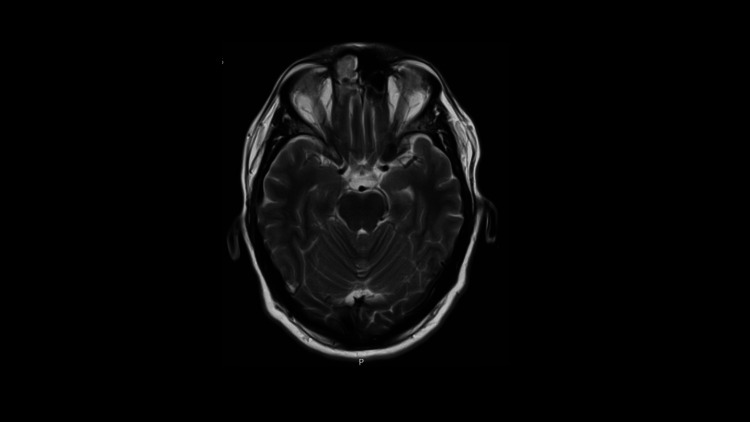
MRI head with contrast showing complete resolution of hyperdense areas with normal enhancement.
